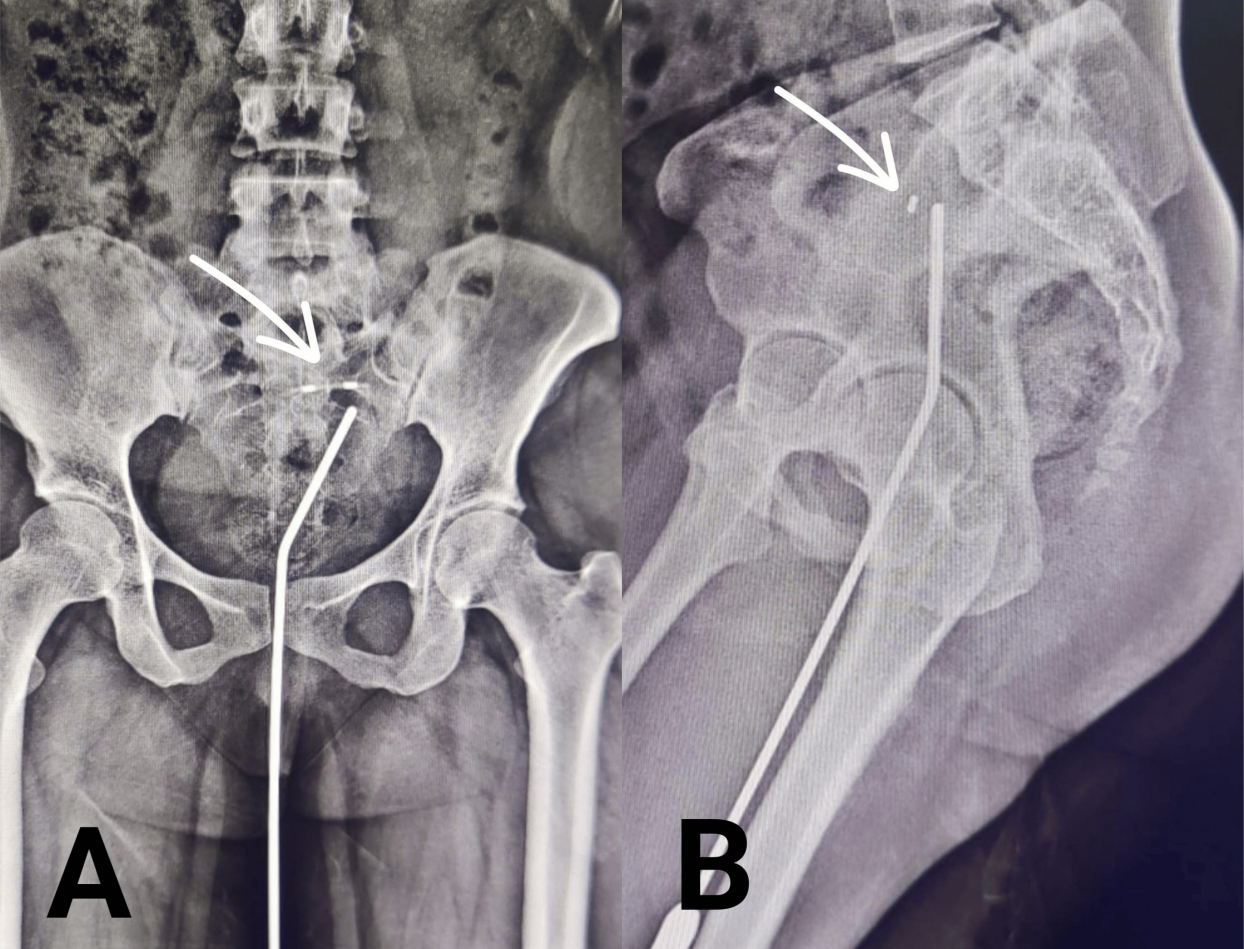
X-ray of the pelvis with uterine sound in situ. (A) Anteroposterior view and (B) lateral view. The location of the intrauterine contraceptive device is marked with white arrows.
